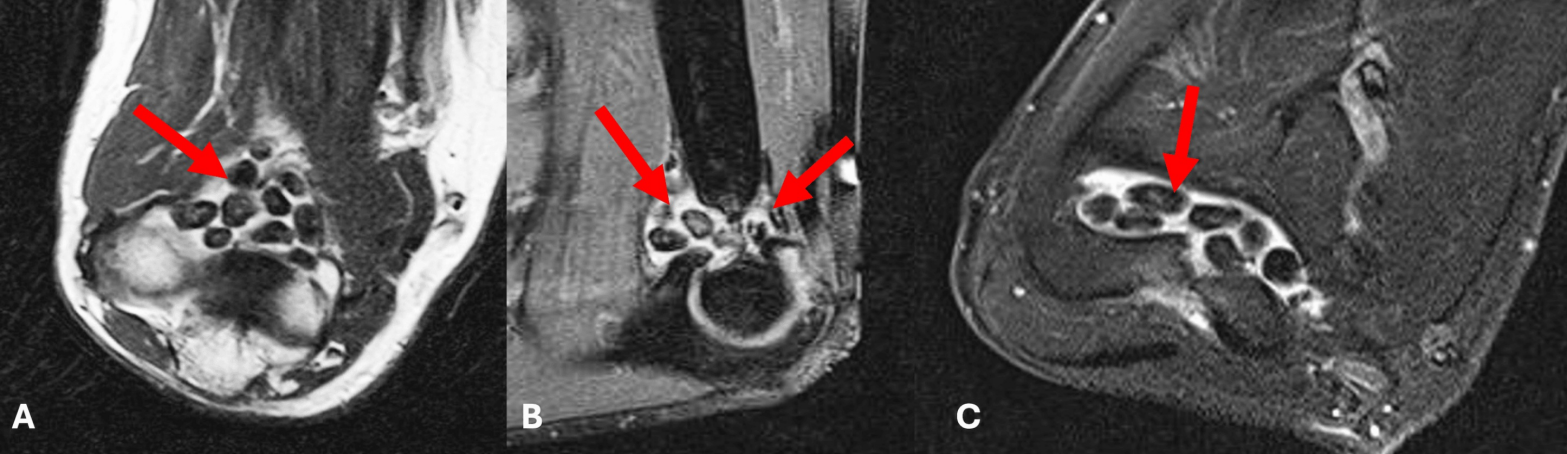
Pre-operative magnetic resonance imaging (MRI) of the right elbow MRI in the (A) coronal, (B) sagittal, and (C) axial planes demonstrate multiple loose bodies in the elbow joint (red arrows).
